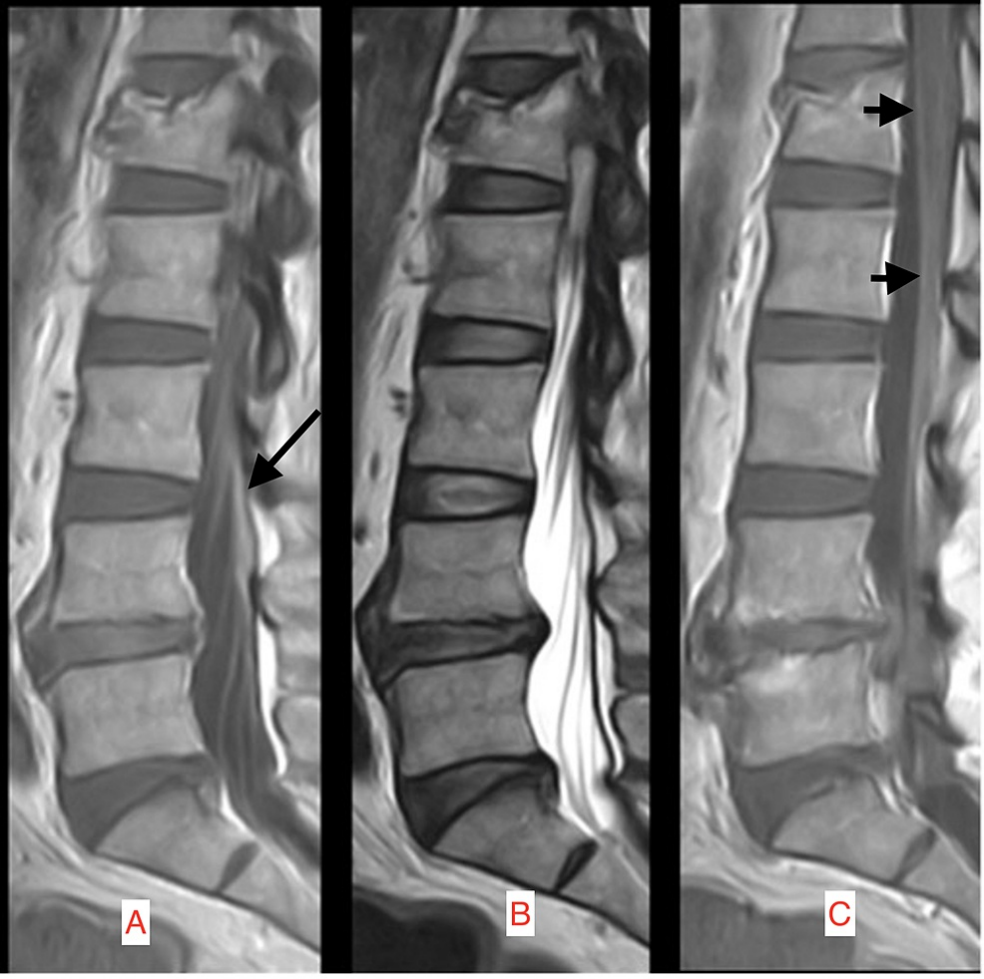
MRI of the spine done three weeks after admission The Magnetic Resonance Imaging (MRI) image is demonstrating a new enhancement of the distal spinal cord. A: Contrast-enhanced T1 sequence sagittal MRI image of the spine demonstrating cauda equina enhancement (long arrow). B: T2 sequence sagittal MRI image of the distal spinal cord corresponding to image A. C: Contrast-enhanced T1 sequence sagittal MRI image of the spine demonstrating lumbar spine enhancement (short arrows).
